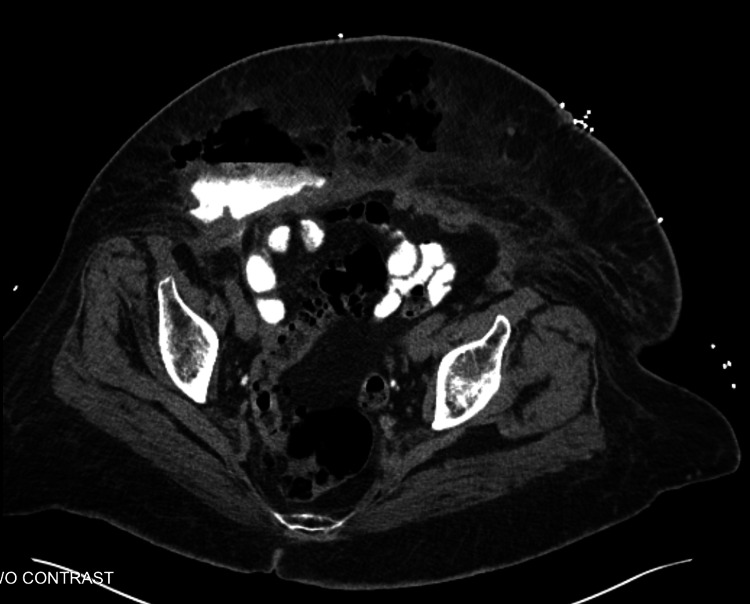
CT abdomen/pelvis with intravenous contrast showing extensive subcutaneous emphysema throughout the ventral abdominal/pelvic wall; a large cavity is seen within the subcutaneous tissues of the right lower quadrant with layering fluid and extravasation of oral contrast that appears to communicate with loops of small bowel in the right lower quadrant consistent with enteric fistula
